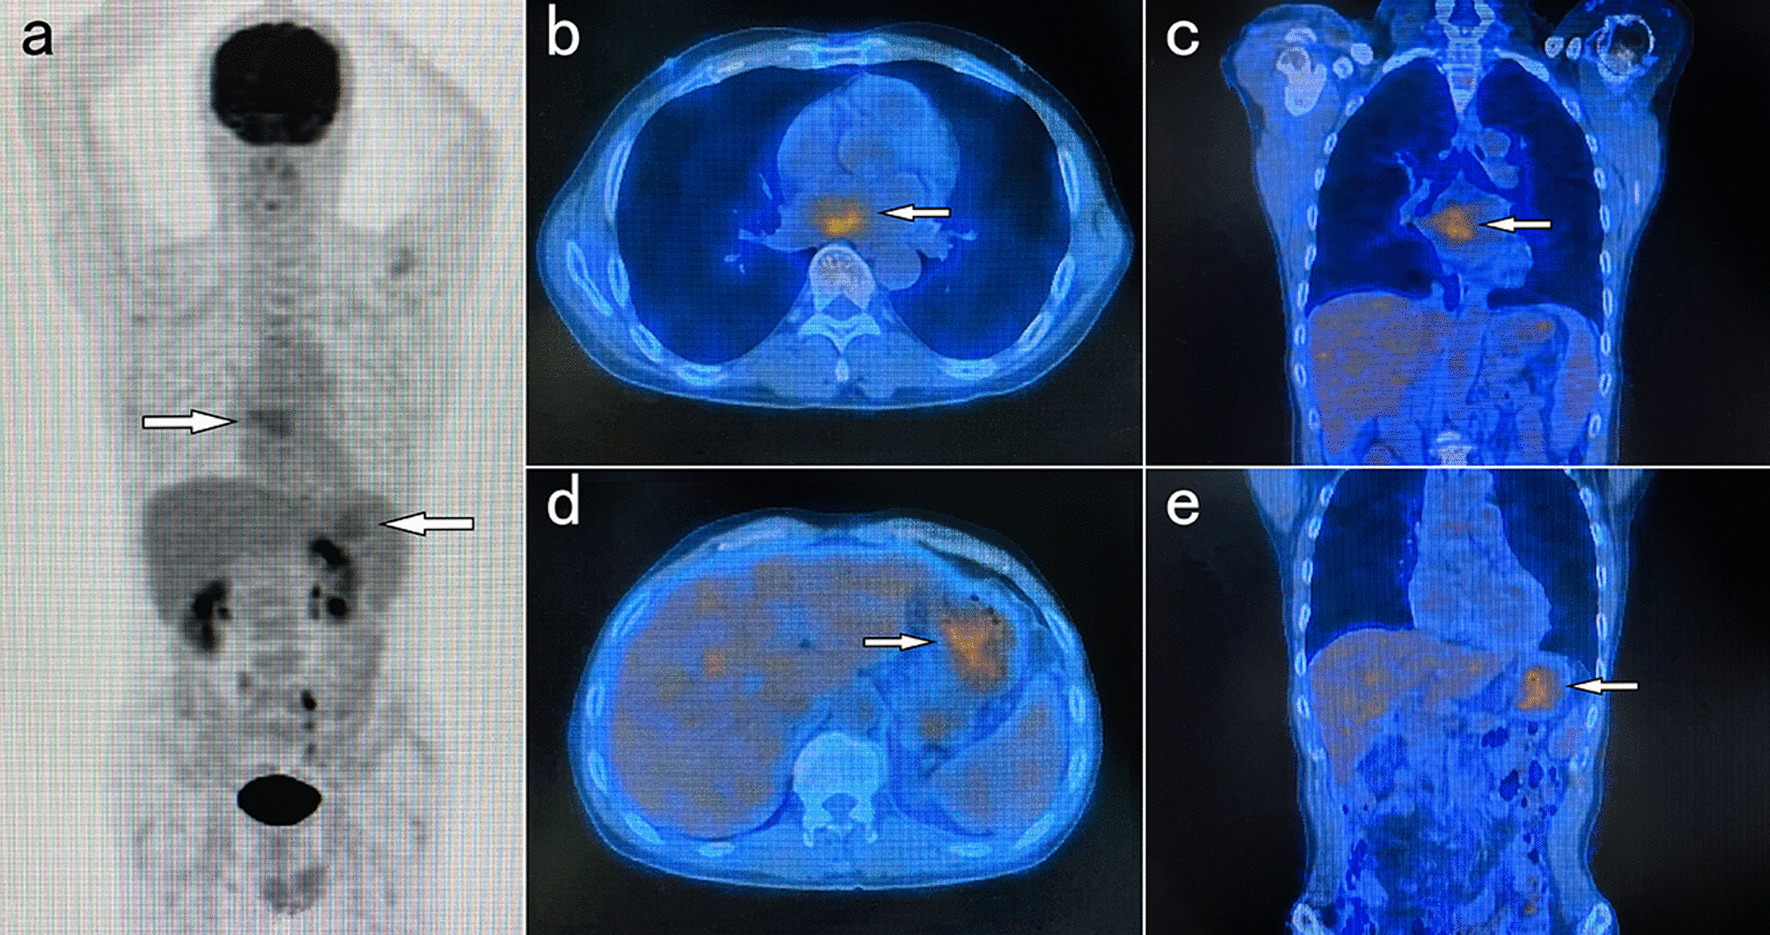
a Maximum-intensity projection images showing increased uptake of radiotracer in the gastric and cardiac regions (arrow); b + c Axial and coronal fusion images showing increased cardiac radiotracer uptake (arrow), placing the region of interest (ROI) in an elevated cardiometabolic shadow with a maximum standardized update value (SUVmax) of approximately 3.1; d + e Axial and coronal fusion images showing increased gastric radiotracer uptake (arrow), with a SUVmax of 3.27 when the ROI was placed in the metabolically elevated shadow of the gastric mucosa adjacent to the gastric mass
